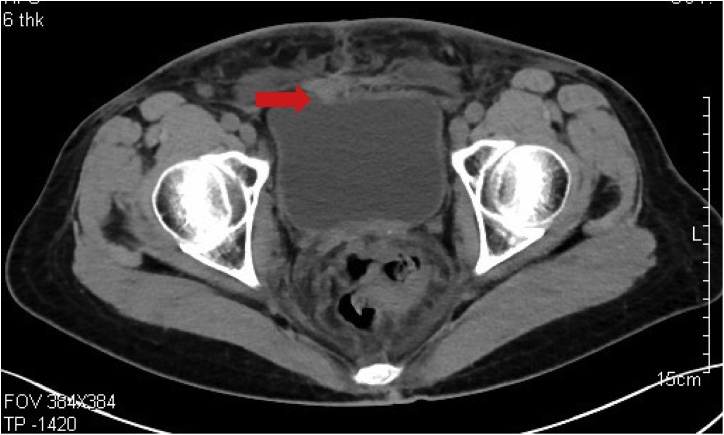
Computed tomography revealed a small lesion on the superior wall of the urinary bladder.
